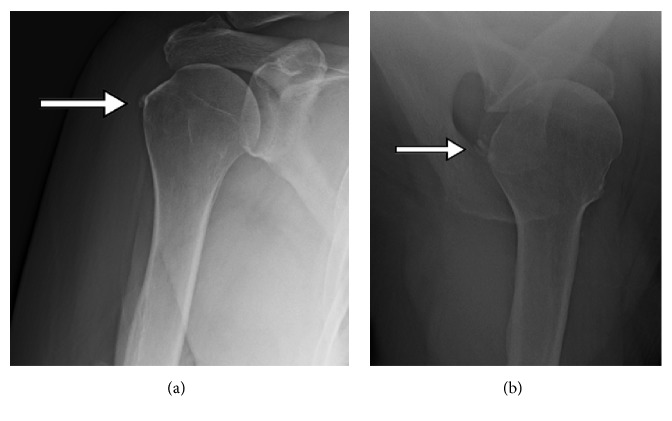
Radiographs with no distinct bone lesions noted. However, subtle decrease in trabecular bone density adjacent to the greater tuberosity is noted in (a) near the arrow tip. The arrows also indicate mild calcific tendinitis.
